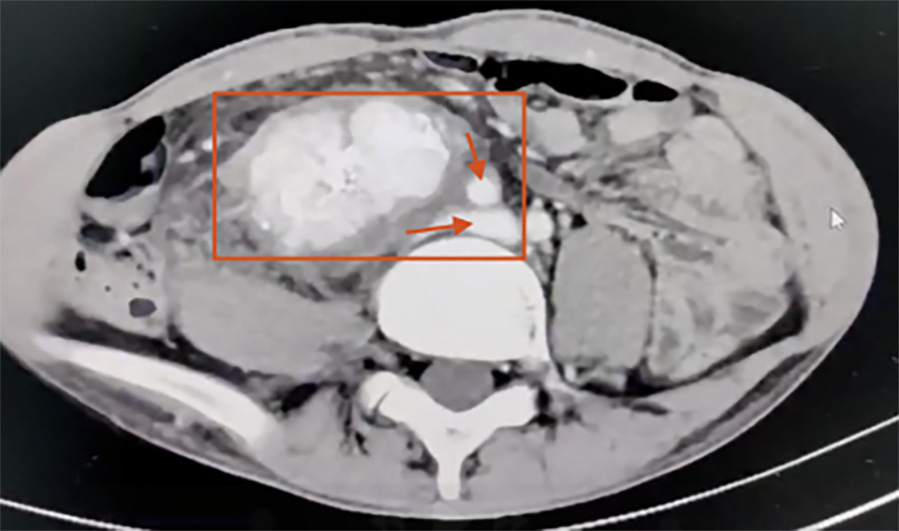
Contrast-enhanced abdominal CT scan demonstrating a malignant retroperitoneal mass (17 × 10 × 4 cm) encasing the right iliac vessels and inferior vena cava (the red box highlights a retroperitoneal malignant mass. The superior arrow indicates encasement of the right iliac vessels, while the inferior arrow indicates encasement of the inferior vena cava).
